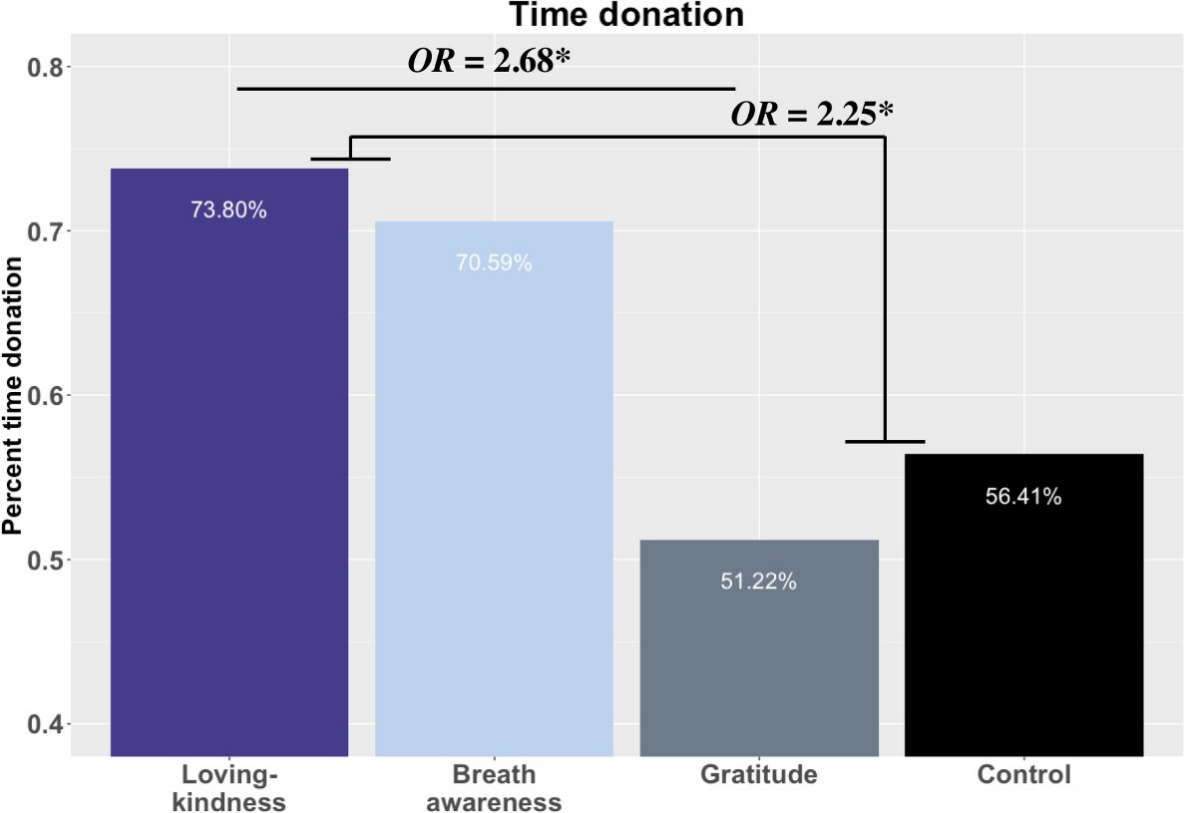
Training effects on generosity. * = *p* < .001, * = p* < .01,  = p <* .05, *t = p* < .10; 4A. Loving-kindness = LK; Breath awareness = BA; Gratitude = GT; Control = CT.
